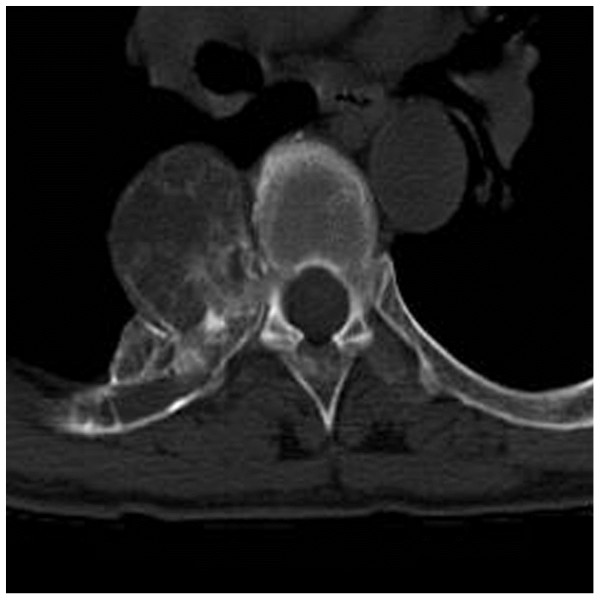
CT reveals a lytic lesion in right fifth posterior rib with ossified matrix.
